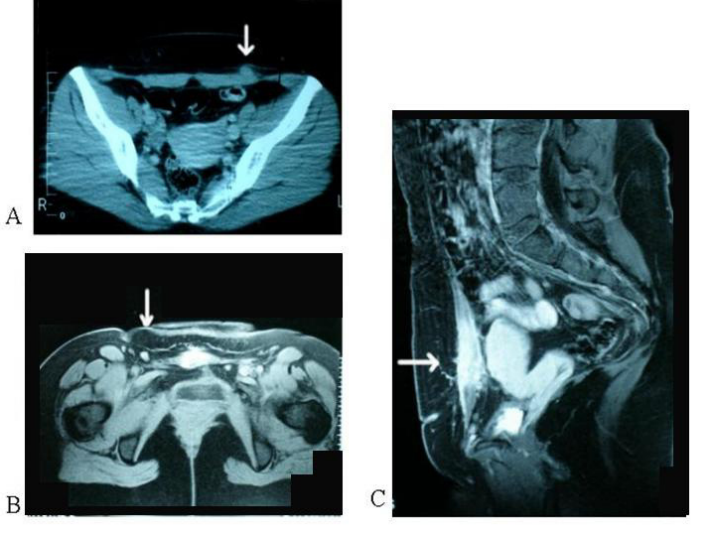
MRI of scar endometriosis nodules. Endometriotic lesions (arrows) appear isodense to muscle on transverse (A, B) and axial (C) T1-weighted spin-echo MRI. A: Case 1; B and C: Case 2.
